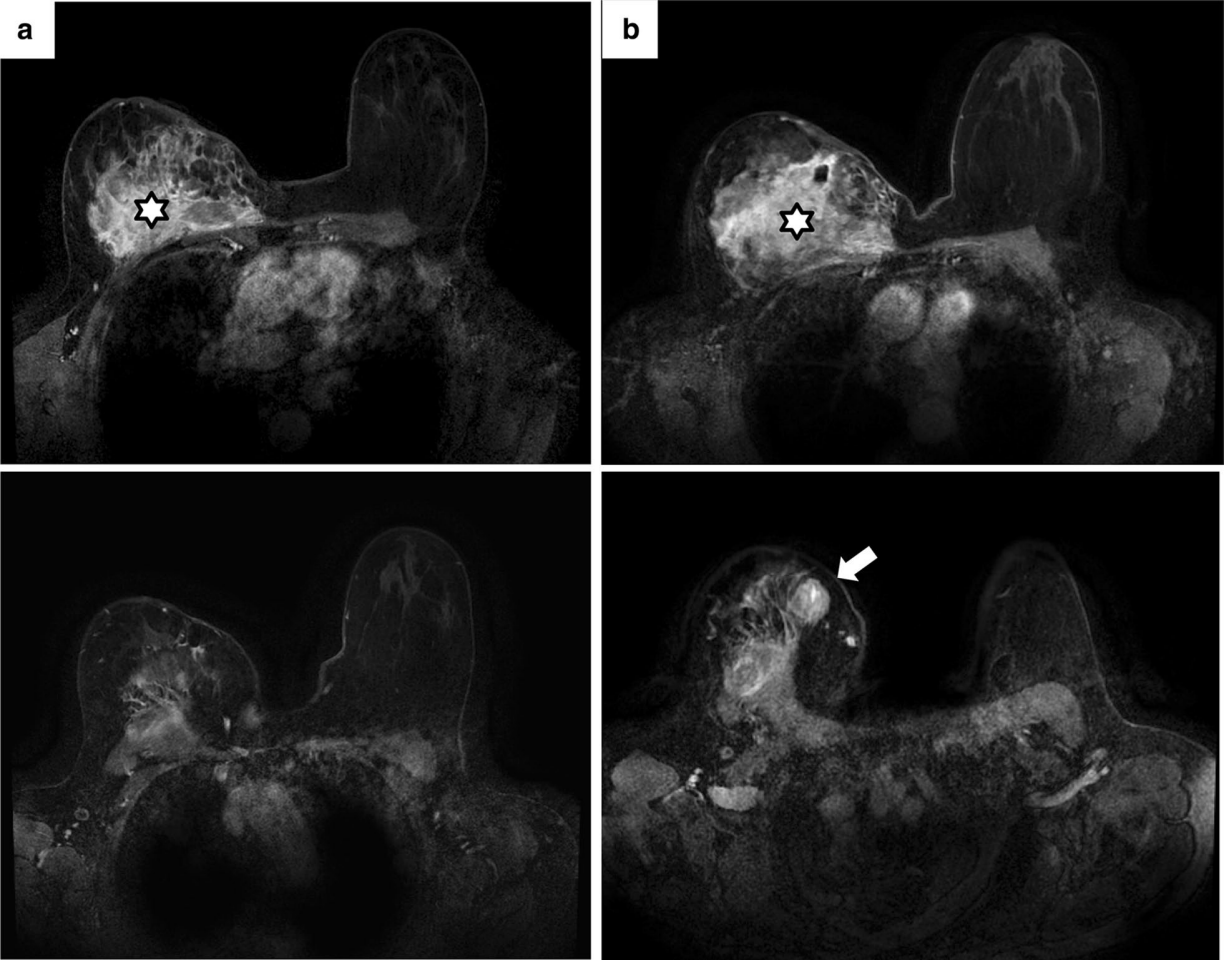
Appearance of a tumor within the desmoid tumor: radiological aspect. Breast T1-weighted Fat Sat MR imaging of middle (*top*) and upper (*bottom*) sections of the *right* breast after gadolinium injection in January 2001 (a) and in January 2013 (b). The desmoïd tumor (*white star*) increased in size between the two dates. Surprisingly, a 2-cm tissue mass (*white arrow*), corresponding to an undifferentiated sarcoma, appeared in the *upper-inner* quadrant within the desmoid tumor in January 2013
